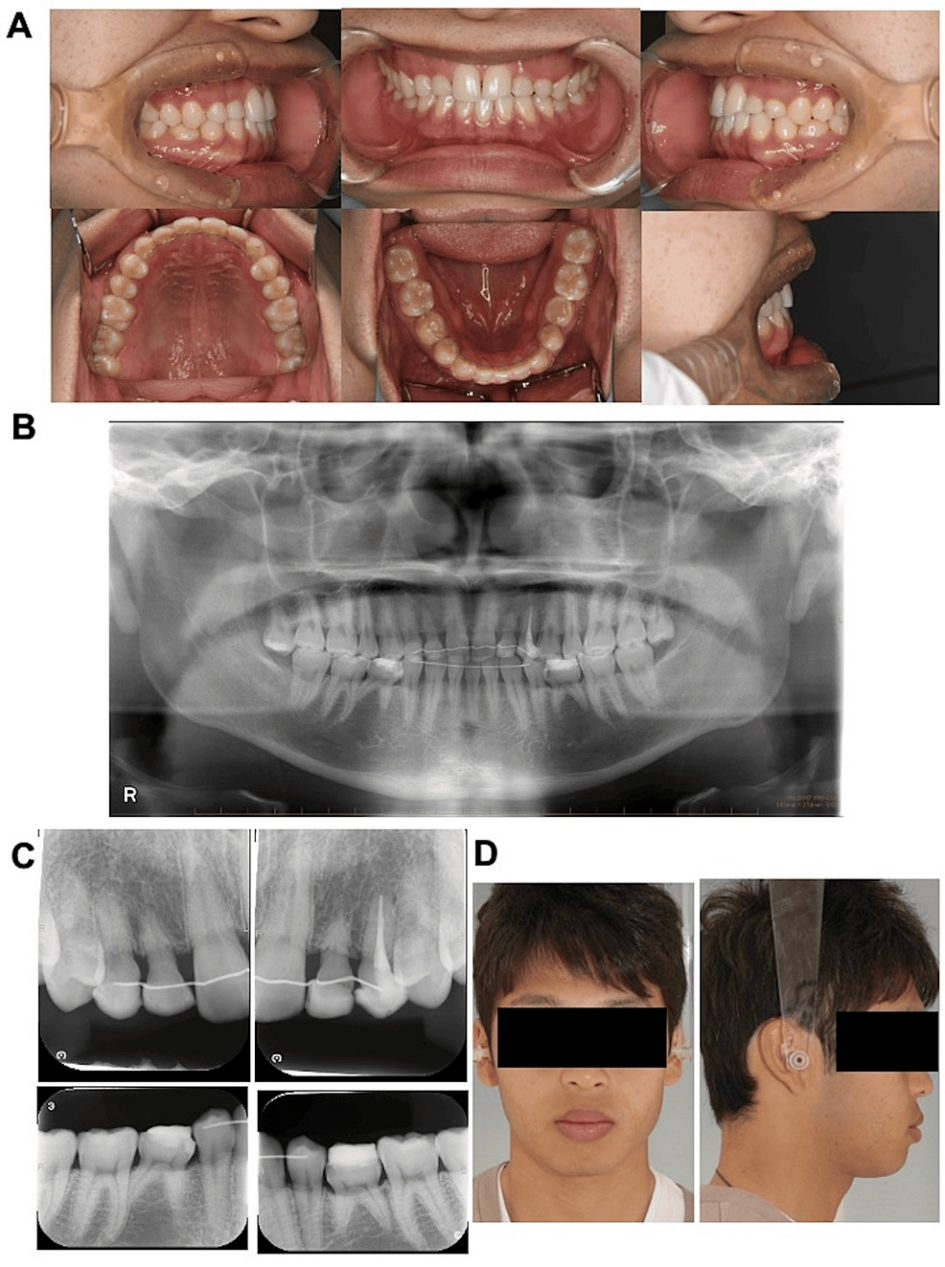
Six years after the retention treatment A: intraoral photographs, B: panoramic radiograph, C: dental radiograph, D: facial photographs
